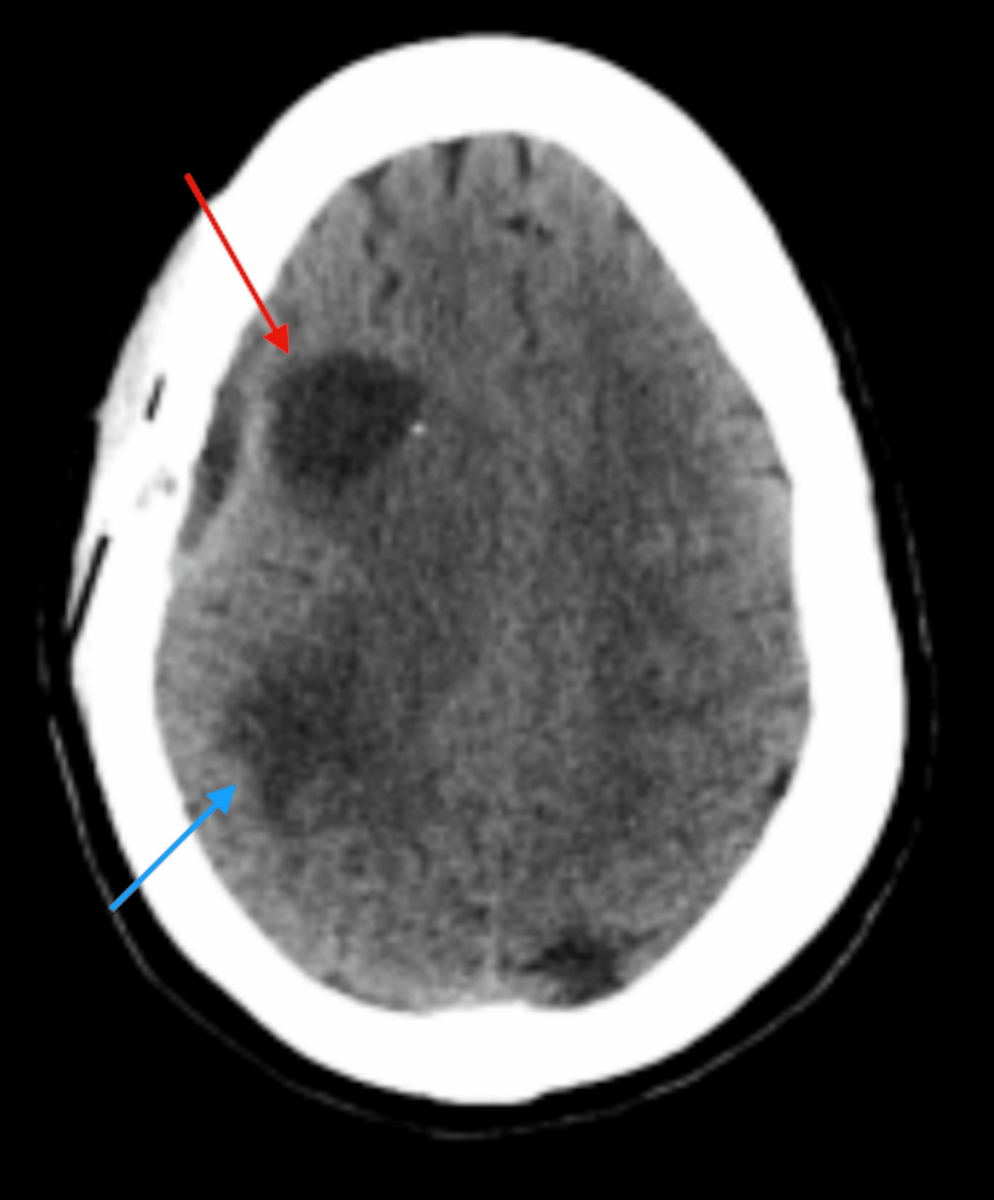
CT of the head revealing a new ill-defined 2.6 cm area of right peritrigonal white matter edema (blue arrow) and post-surgical changes with a 2.3 x 2.6 x 2.4 cm fluid collection in the right frontal resection cavity (red arrow).
